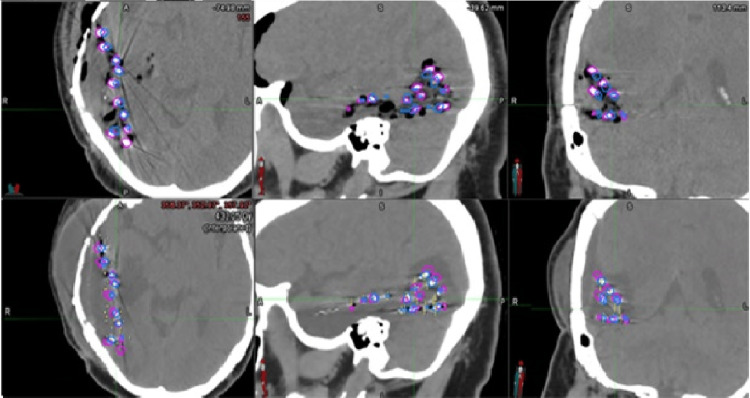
Seed positions identified on day one and day 30 post-implant CT images superimposed on both CT images. Significant maximum seed displacement was observed in a few cases. Seed positions identified on day one and day 30 post-implant CT images superimposed on day one and day 30 post-implant CT images for a patient are shown here as an example. Day one CT is shown in the top row while day 30 CT is shown in the bottom row with seeds of day one shown in pink and day 30 in blue, overlaying in both image sets.
